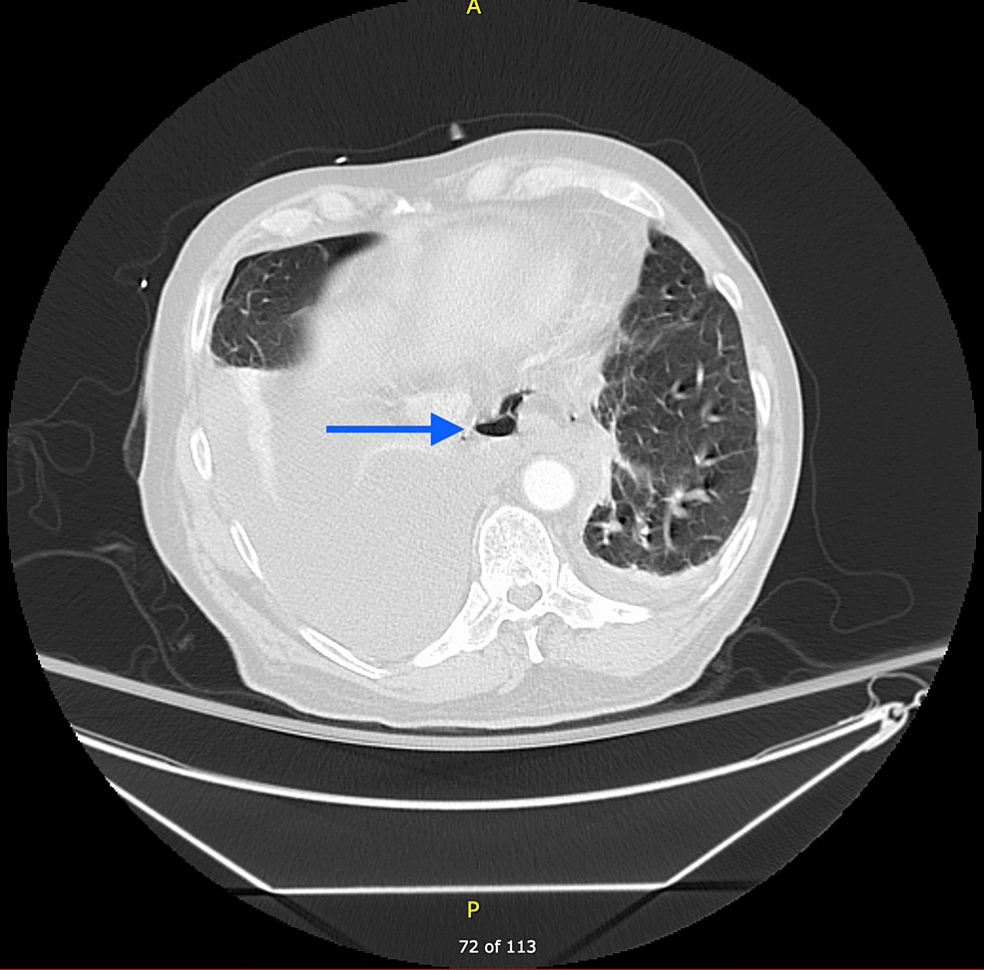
CT chest with contrast showing esophageal thickening with adjacent pneuma-mediastinum.
